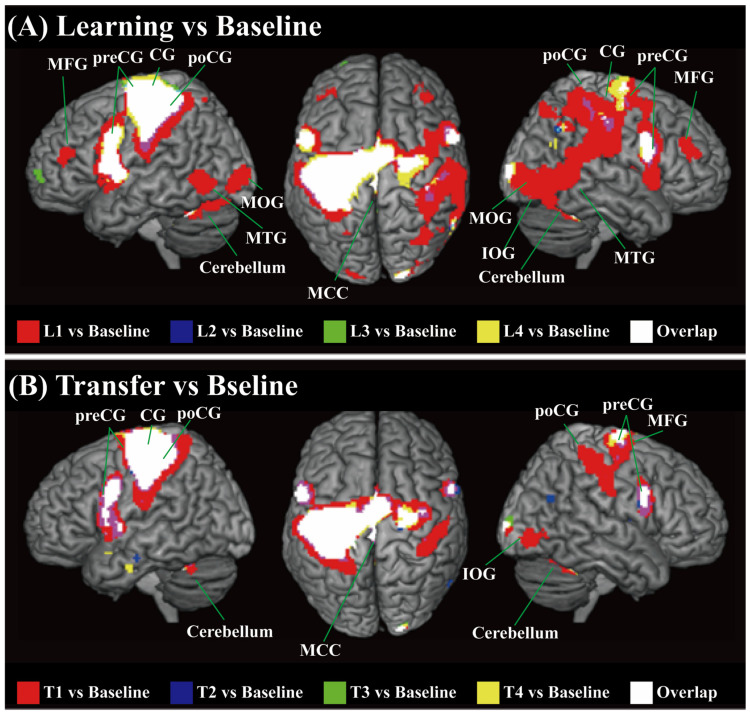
Cortical activation patterns in (A) the learning period vs. the baseline period and (B) the transfer period vs. the baseline period were superimposed on surface-rendered high-resolution MR images unrelated to the participants in the present study, viewed from the left, top, and right of the brain. Regions in white were activated during all tasks (overlap). The significance threshold was set at *p* < 0.05, which was corrected for multiple comparisons (FWE) across the whole brain. SFG: superior frontal gyrus; MFG: middle frontal gyrus; SMG: supramarginal gyrus; CG: central sulcus; PoCG: postcentral gyrus; preCG: precentral gyrus; MCC: middle cingulate cortex; MOG: middle occipital gyrus; IOG: inferior occipital gyrus; MTG: middle temporal gyrus.
